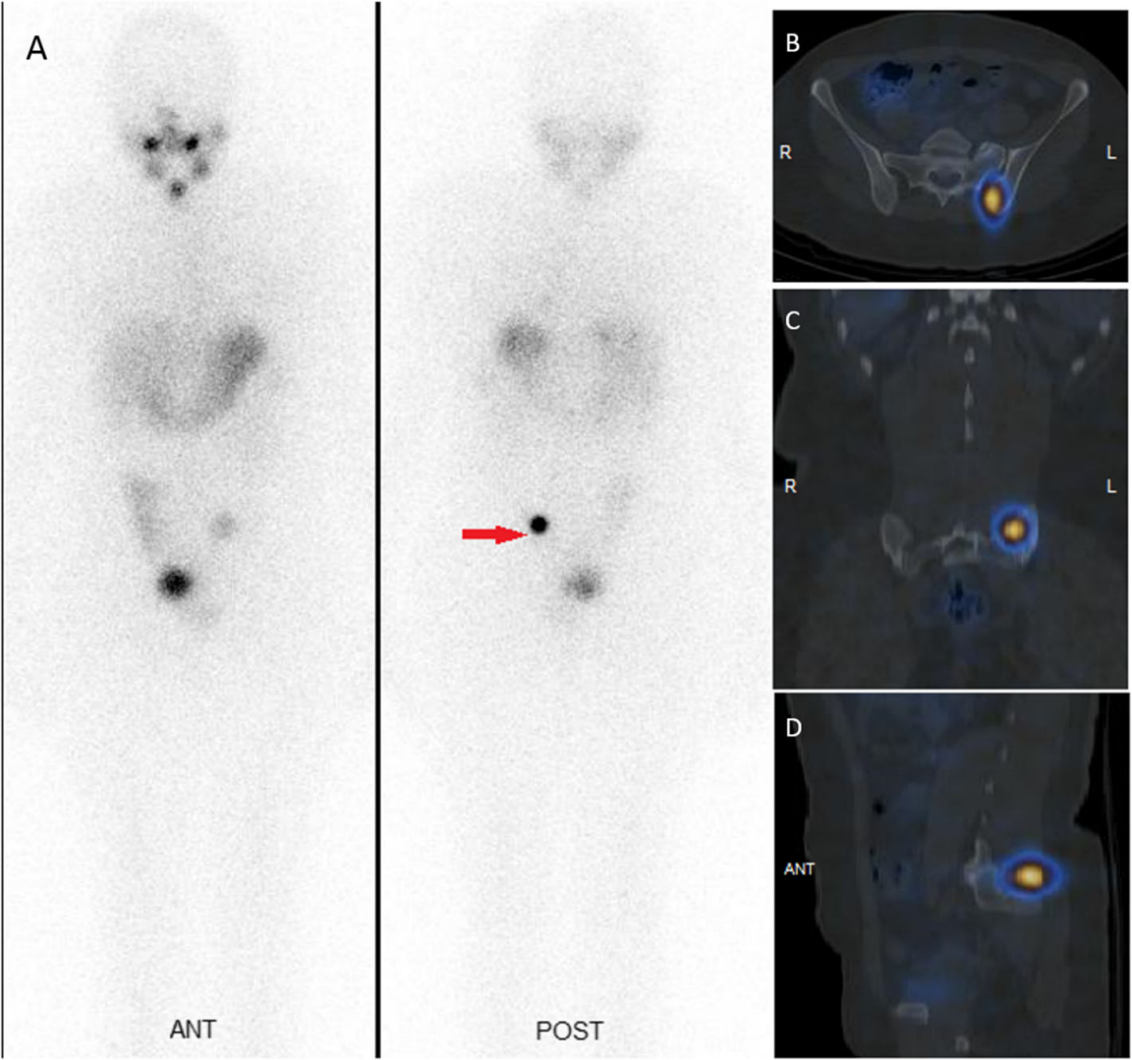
Post ablation whole body iodine scan (A) demonstrates increased focus of iodine uptake in the left hemipelvis and SPECT/CT of the pelvic region (B-D) localized the activity to the left iliac bone
